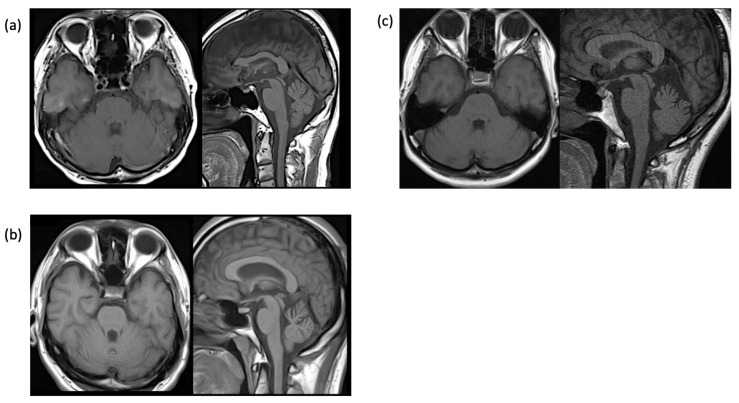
Brain MRI findings of the three patients. (a) Case 1, (b) Case 2, (c) Case 3. T1-weighted axial and sagittal images show mild upper cerebellar vermian atrophy.
